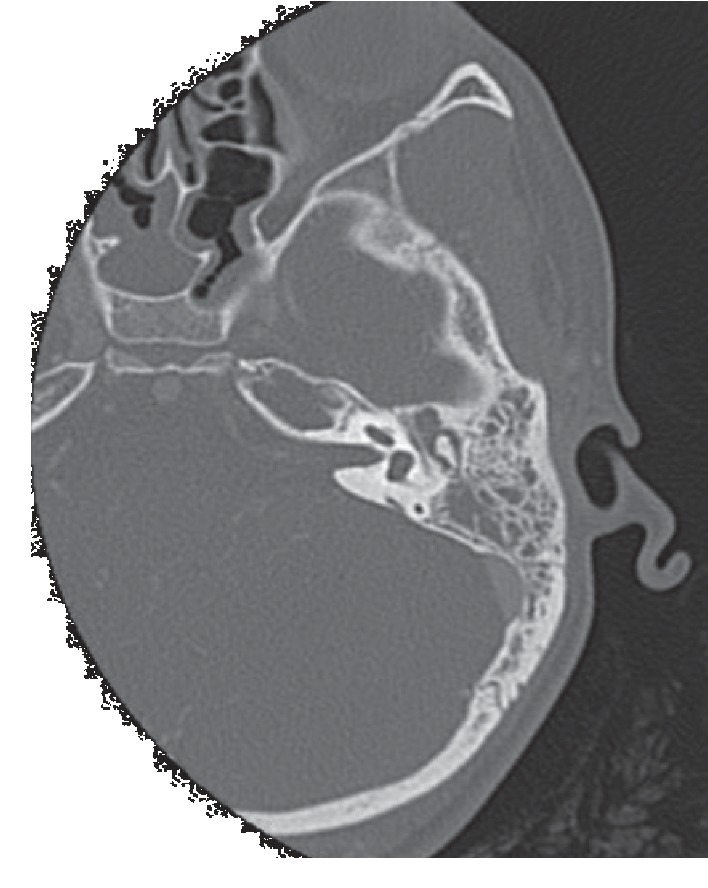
Axial temporal bone computed tomography (CT) scan showing opacification of the left middle ear and mastoid and expanded fluid-filled petrous apex.
